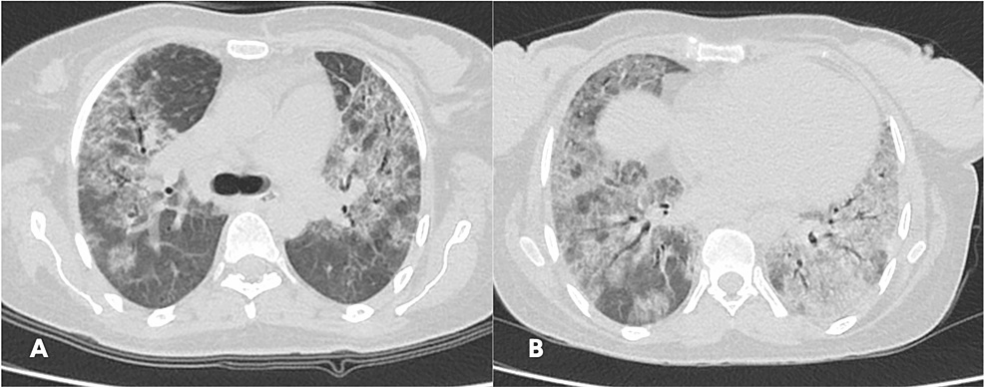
Contrast-enhanced CT scan demonstrating ground-glass opacities and diffuse septal thickening (A, B) with a basal predominance (B).
